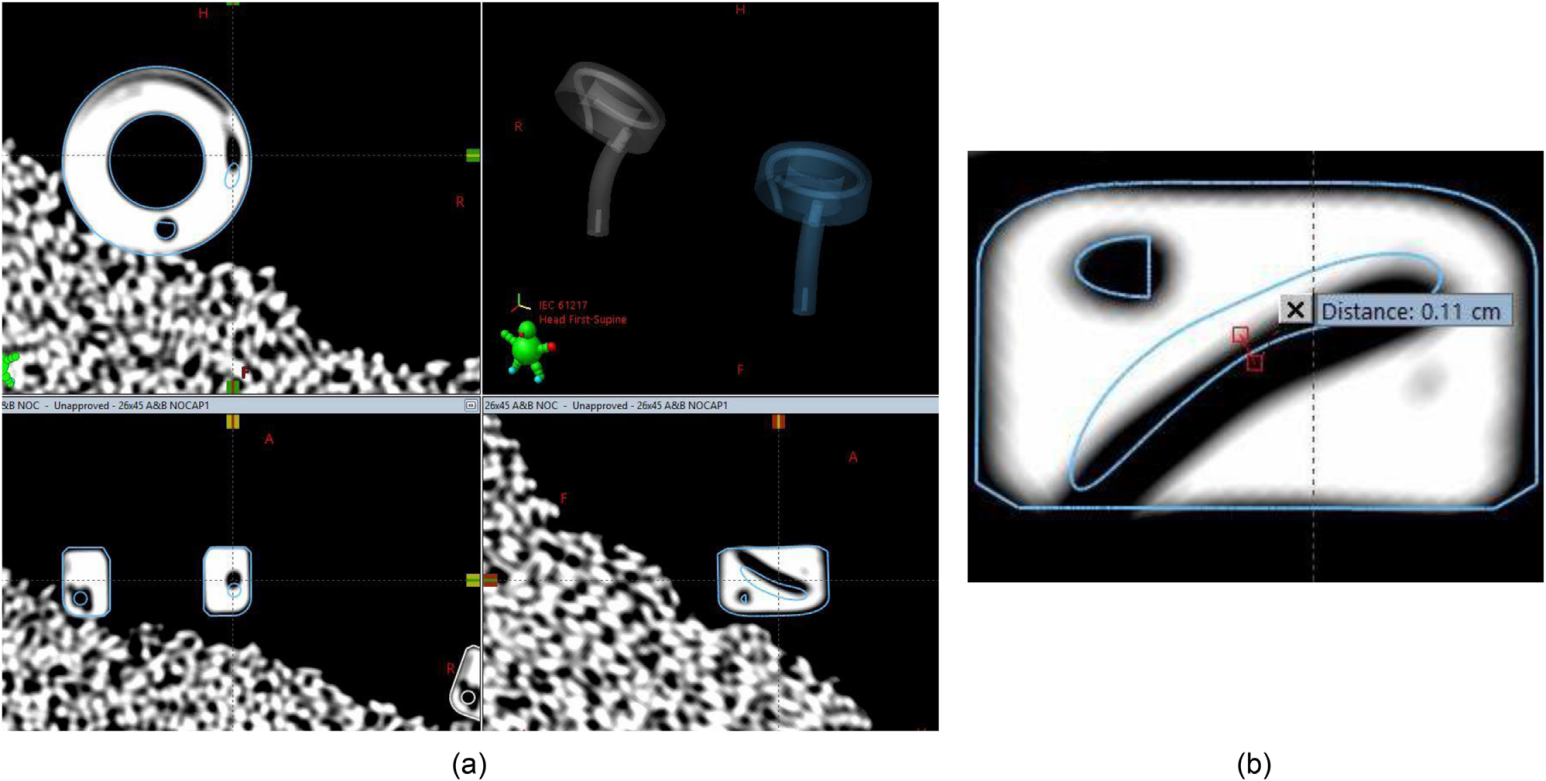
CT images viewed in the treatment planning system of a 45° ring set, ⌀26 mm × 32 mm with no ring cap and no marker wire in place in all three planes in (a) and magnified with distance indicated in (b). The manufacturer‐provided digital 3D model is overlaid with the CT data. The center of the source channel out of the ring plane in the solid model is displaced from the corresponding position in the CT data by approximately 1 mm.
